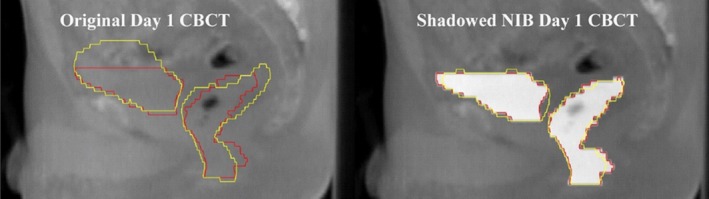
Contour Propagation Model example. Left: Original CBCT images data for the first fractional treatment of a prostate cancer patient treated with the Versa system. Contours of the bladder and rectum shown in red are the contours manually delineated in the CBCT. Contours in yellow are NIB contours propagated from the planning CT structures. Right: The same CBCT image data following contrast enhancement via the manually drown contours (red) that enables the shadowed NIB contour propagation (yellow).
